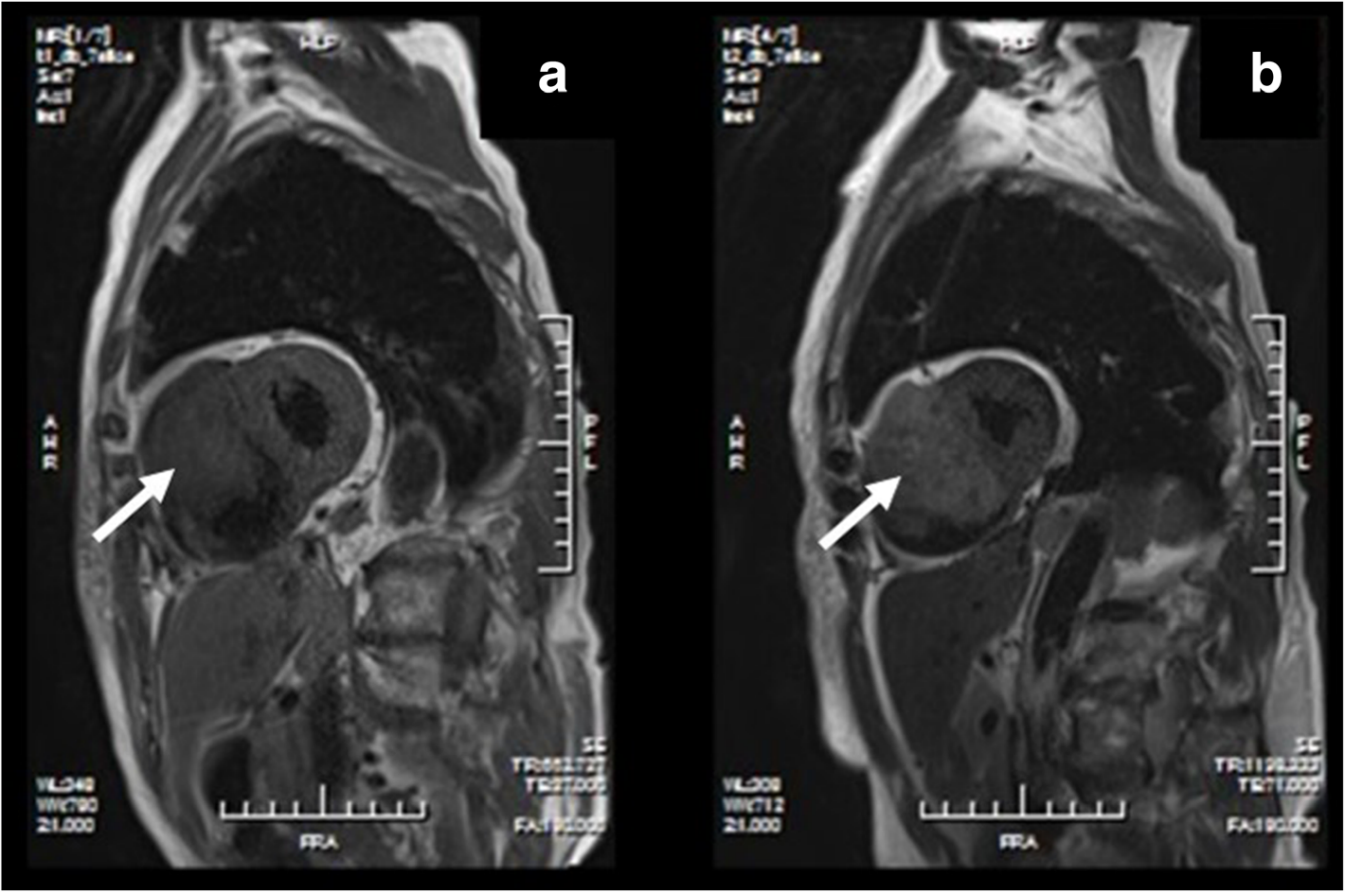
Cardiac magnetic resonance imaging studies. a and b The presence of the tumor was confirmed by cardiac magnetic resonance imaging, which showed a mass occupying all the space in the right ventricle. The tumor and interventricular septum were poorly marginated. The *arrows* indicate tumors
